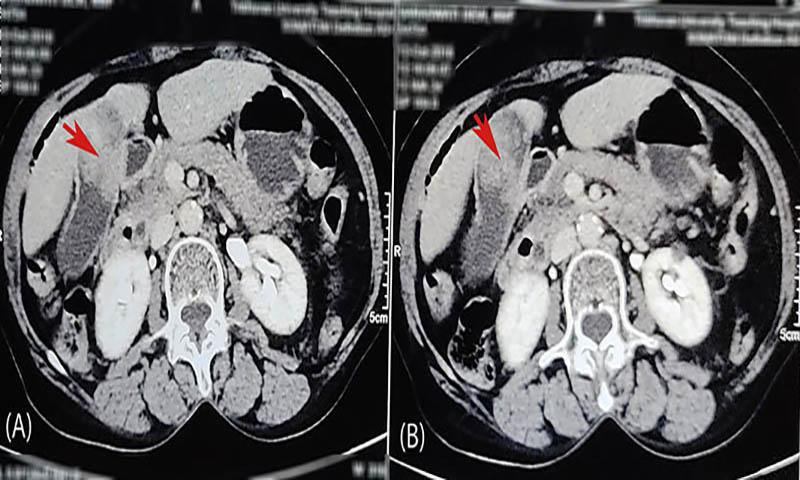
A, B) Contrast-enhanced CT scan of abdomen-pelvis done two years back showing enhancing mass in the neck of the gallbladder (shown by the red arrows).
